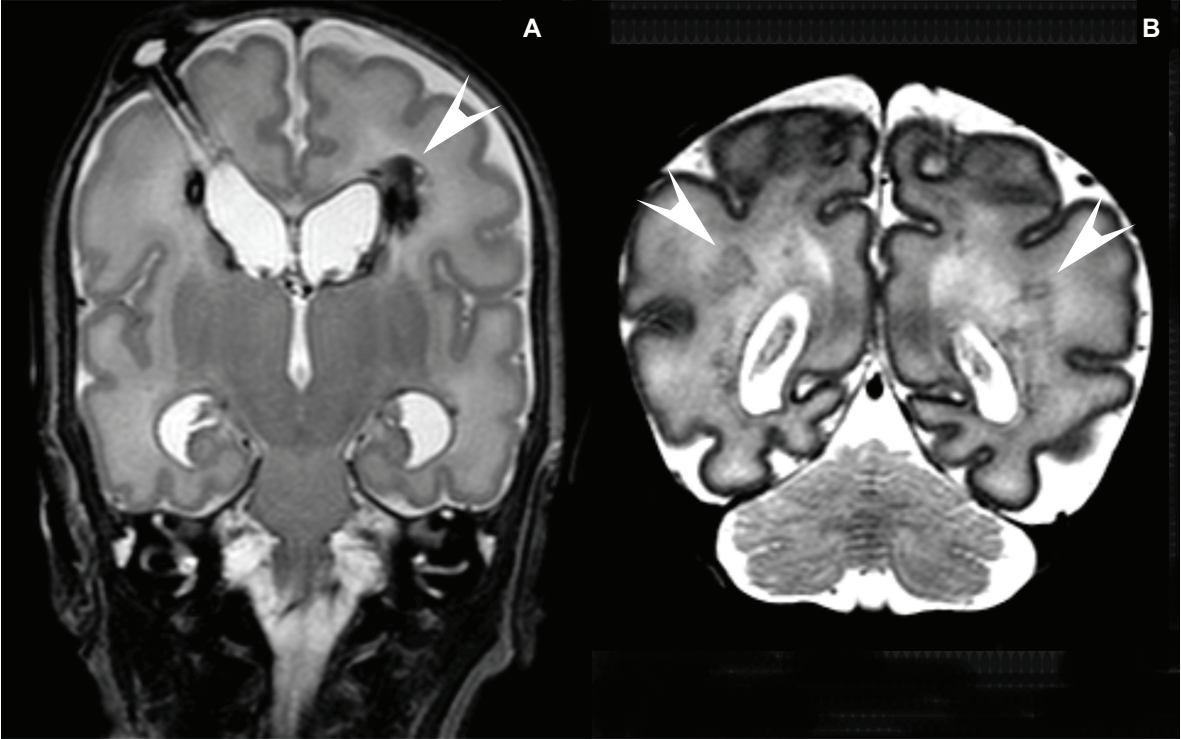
(A) MRI of a preterm infant (gestational age 26 2/7 weeks, birthweight 965 g) with a large IVH and a large left-sided (arrowhead) and small right-sided venous infarct. Post-hemorrhagic ventricular dilatation was treated with CSF removal from a subcutaneous reservoir. T2 weighted coronal image at the age of 8 weeks after birth. (B) MRI of a preterm infant (gestational age 32 2/7 weeks, birthweight 1,670 g) with several lesions in the periventricular white matter (arrowheads). T2 weighted coronal image at the age of 6 days after birth.
